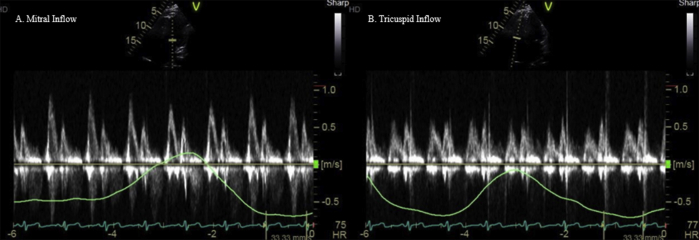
Pulsed-wave-Doppler recordings of (A) mitral (B) tricuspid inflow velocities, with simultaneous respirometric recording, showing no significant respirophasic variations of mitral and tricuspid inflows.
